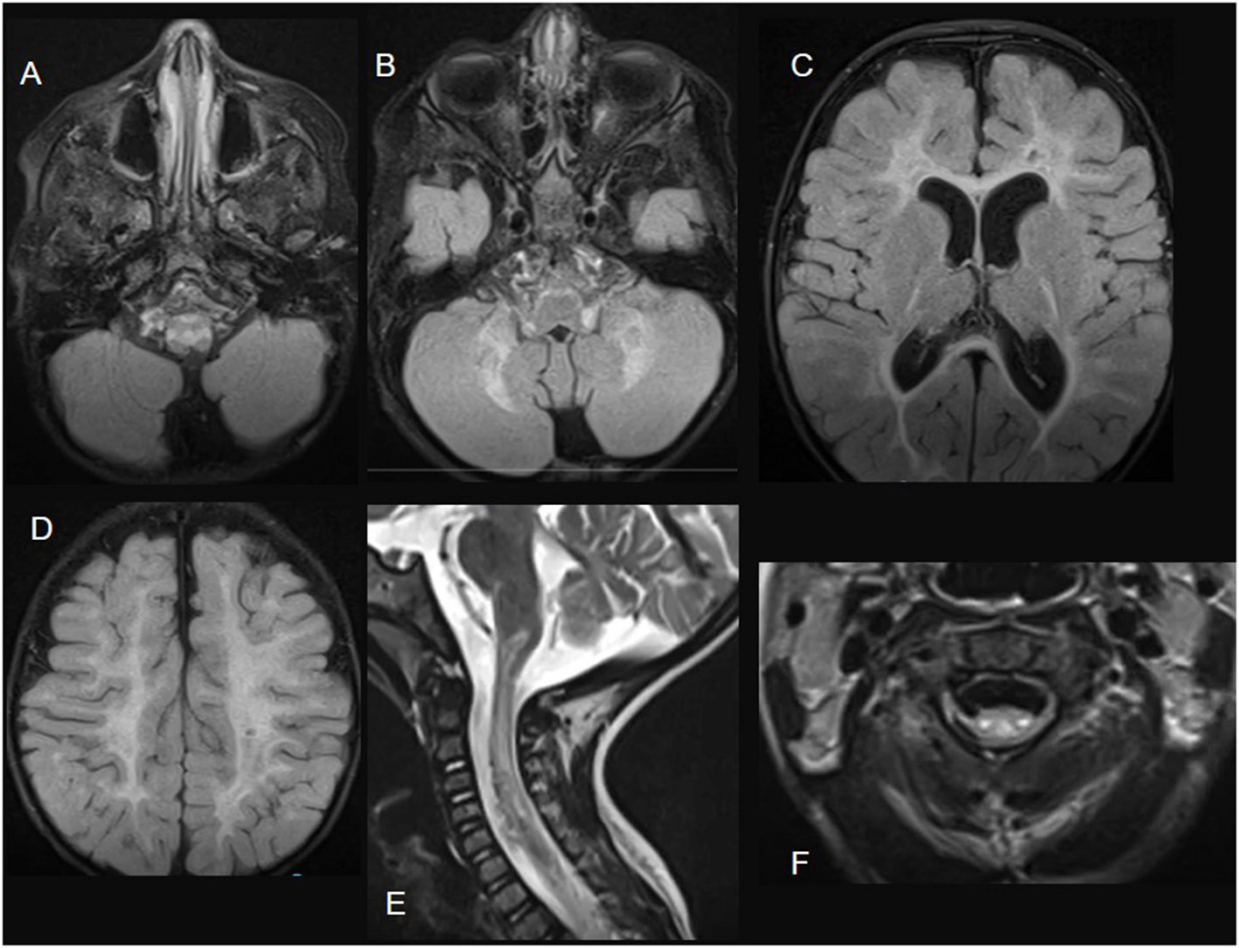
ISCA2*-related disease. Brain MRI images; (A–D) axial FLAIR sections showing extensive diffuse bilateral supra and infratentorial signal abnormalities involving subcortical, deep, and subcortical white matter. Sagittal (E) and axial (F) T2-weighted images demonstrate spinal cord white matter involvement of the dorsal columns and the lateral corticospinal tracts.
